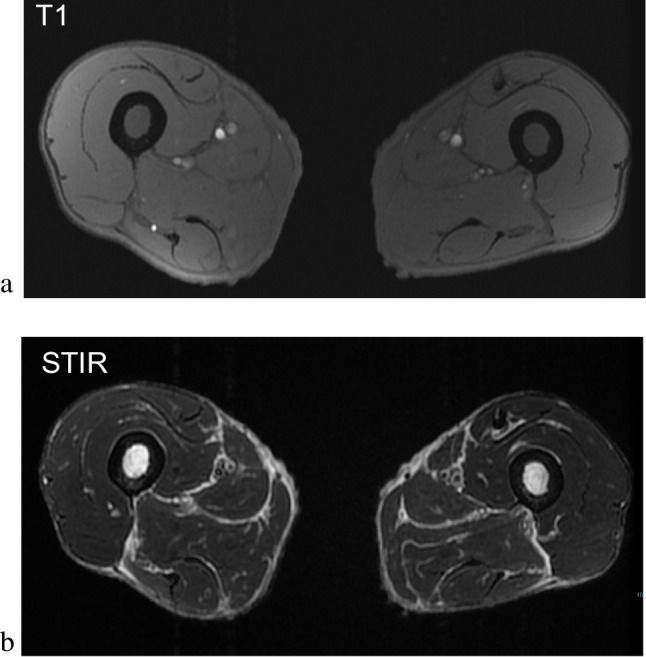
SABM in a 45-year-old male with anorexia nervosa and rapid weight loss. T1-weighted sequences (a) and STIR sequences (b) demonstrate diffuse loss of the subcutaneous and intermuscular fat in SABM. Residual visible fat shows abnormal intermediate to hypointense T1 signal and hyperintense fluid sensitive signal. Hypointense T1-weighted marrow signal in (a) and STIR hyperintense marrow signal in (b) is also consistent with SABM
